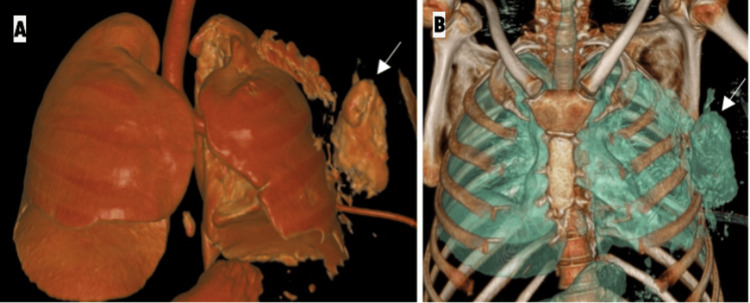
Volume-rendered three-dimensional reconstructed CT images (A and B) show the intercostal lung hernia through the left fourth intercostal space (white arrow).
